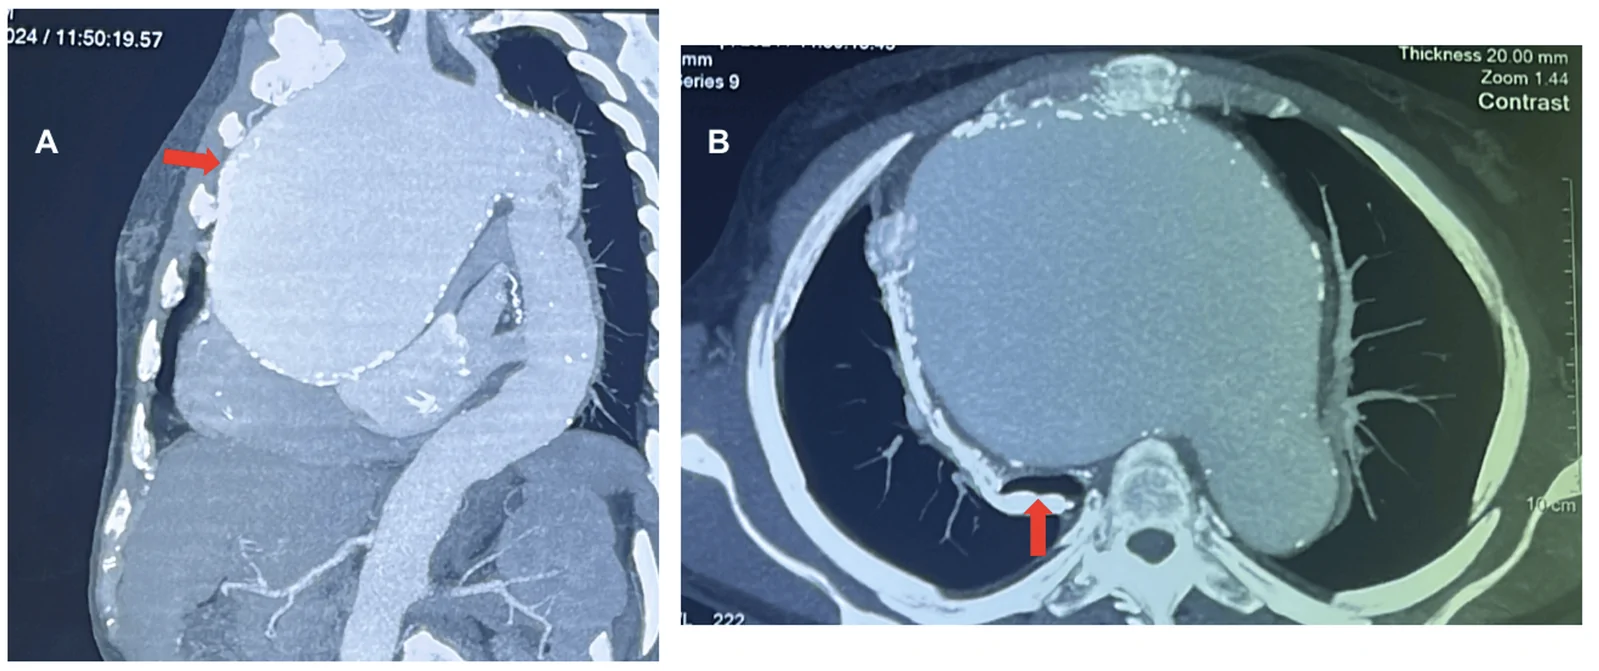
Preoperative contrast-enhanced computed tomography (CECT) (A) Sagittal reconstruction showing a giant ascending and arch aortic aneurysm (red arrow). (B) Axial section demonstrating tracheal compression and deviation (red arrow) by the aneurysm.
